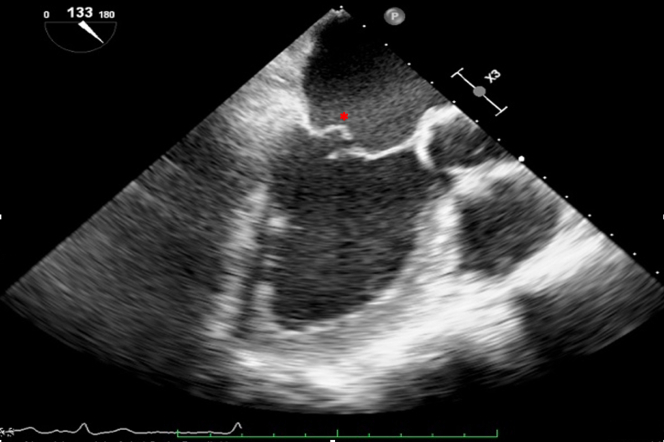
Preoperative Transesophageal Echocardiography Biplane View A cut plane through the medial (A3-P3) part of the valve showing prolapsing posterior mitral valve leaflet tips (red asterisk).
